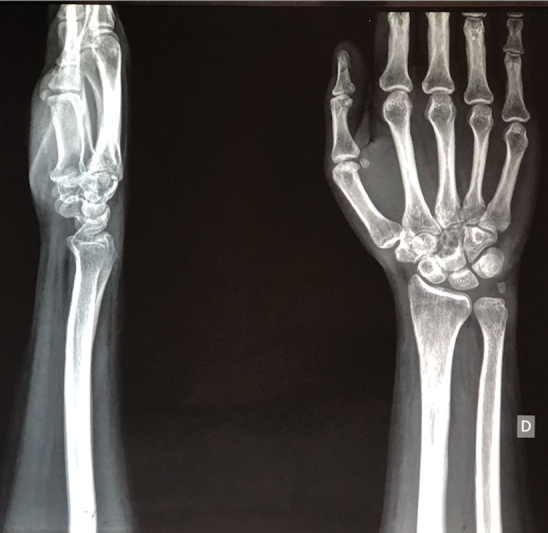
Initial radiograph of the right wrist, revealing a well-defined osteolytic lesion at the capitate bone.
